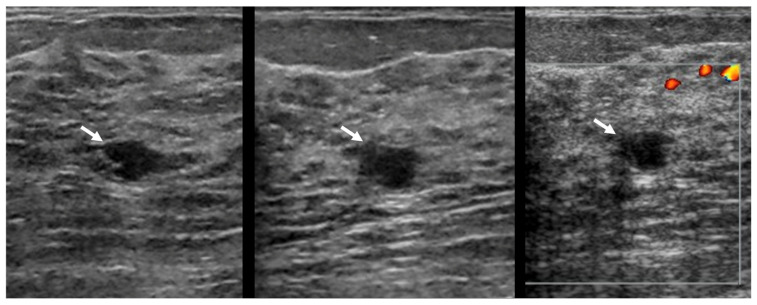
False-negative AI assessment of TNBC. Targeted sagittal (left), transverse (middle), and transverse power Doppler (right) US images directed to a suspicious enhancing mass on MRI in this 48-year-old woman show an oval, circumscribed, anechoic 6-mm mass (arrows) with posterior enhancement and no internal vascularity. This was assessed as BI-RADS 4B due to the context of a correlating enhancing mass on MRI, but, in the validation set, the KDS score was 0.27 (a probably benign rating). Core biopsy showed grade 3 TNBC.
